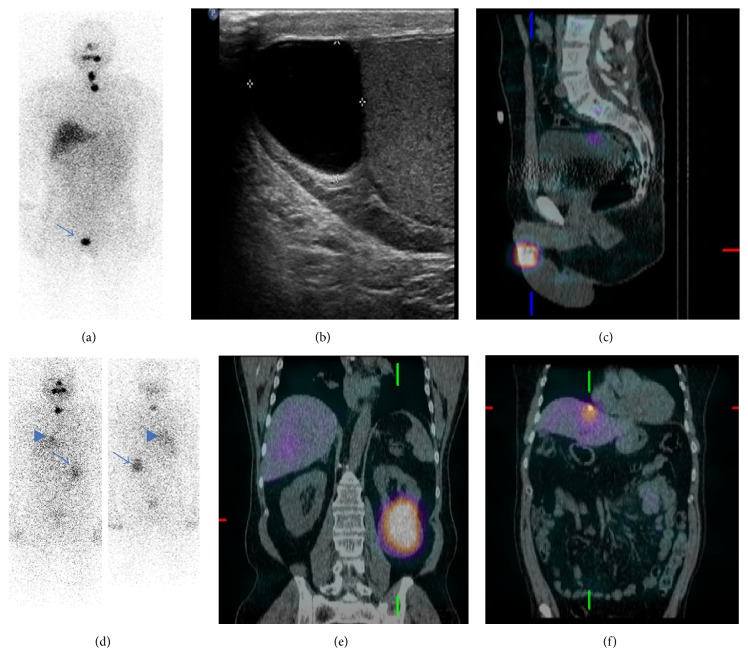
(a) Anterior planar view from a postablation I-131 WBS shows satisfactory targeting of radiotracer in the neck with an additional incidental focus of activity in the region of the right scrotum. (b) Sagittal sonographic image of the right scrotum shows a hypoechoic avascular lesion in the right epididymal head likely representing a spermatocele. (c) SPECT/CT localizes the area of radioiodine uptake to the right epididymal lesion. (d) Anterior and posterior planar views from a postablation I-131 WBS show expected radiotracer uptake in the neck as well as two incidental foci projecting over the dome of the liver (arrowhead) and in the left lower quadrant of the abdomen (arrow). (e) SPECT/CT localizes the area of radioiodine uptake to an exophytic cyst arising from the inferior pole of the left kidney. (f) SPECT/CT localizes the second radioiodine avid focus to a calcified granuloma in the dome of the liver.
